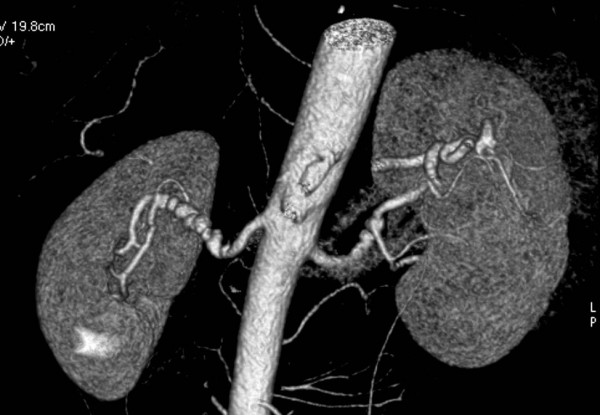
Computed tomography angiography in a patient with medial dibromuscular dysplasia.
